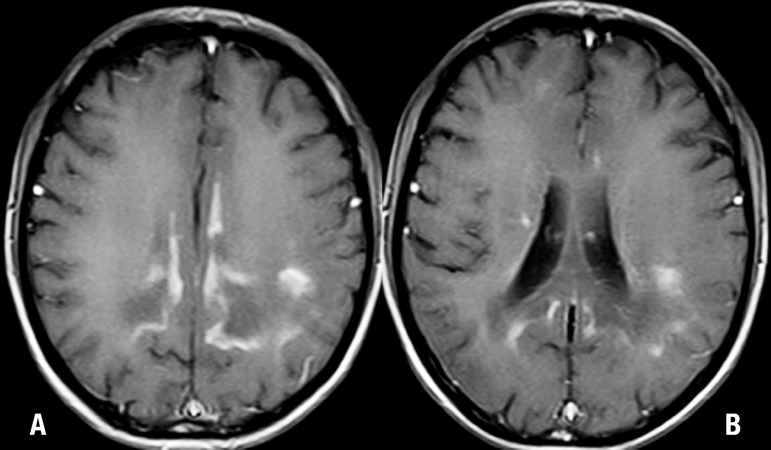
[A, B] Axial post-contrast T1-weighted MR images show
